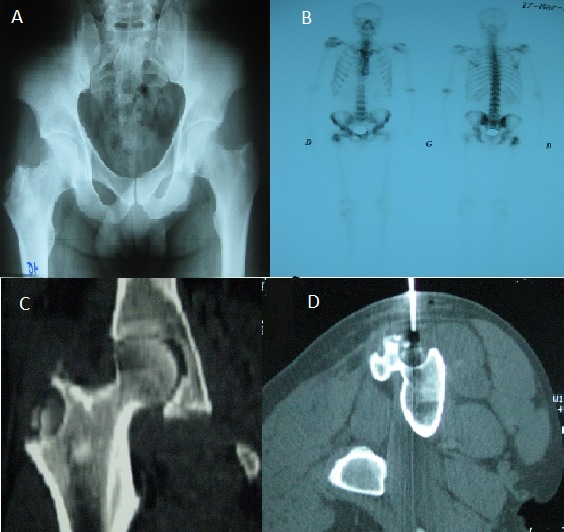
A): radiographie du bassin ; lésion ostéolytique du grand trochanter droit avec épaississement des parties molles en regard; B): scintigraphie osseuse; hyperfixation du grand trochanter droit; C): scanner du bassin: lésion ostéolytique du grand trochanter droit contenant de multiples séquestres, avec irrégularité corticale et collection multicloisonnée en regard de la lésion.; D): ponction-biopsie osseuse du grand trochanter sous contrôle scannographique
